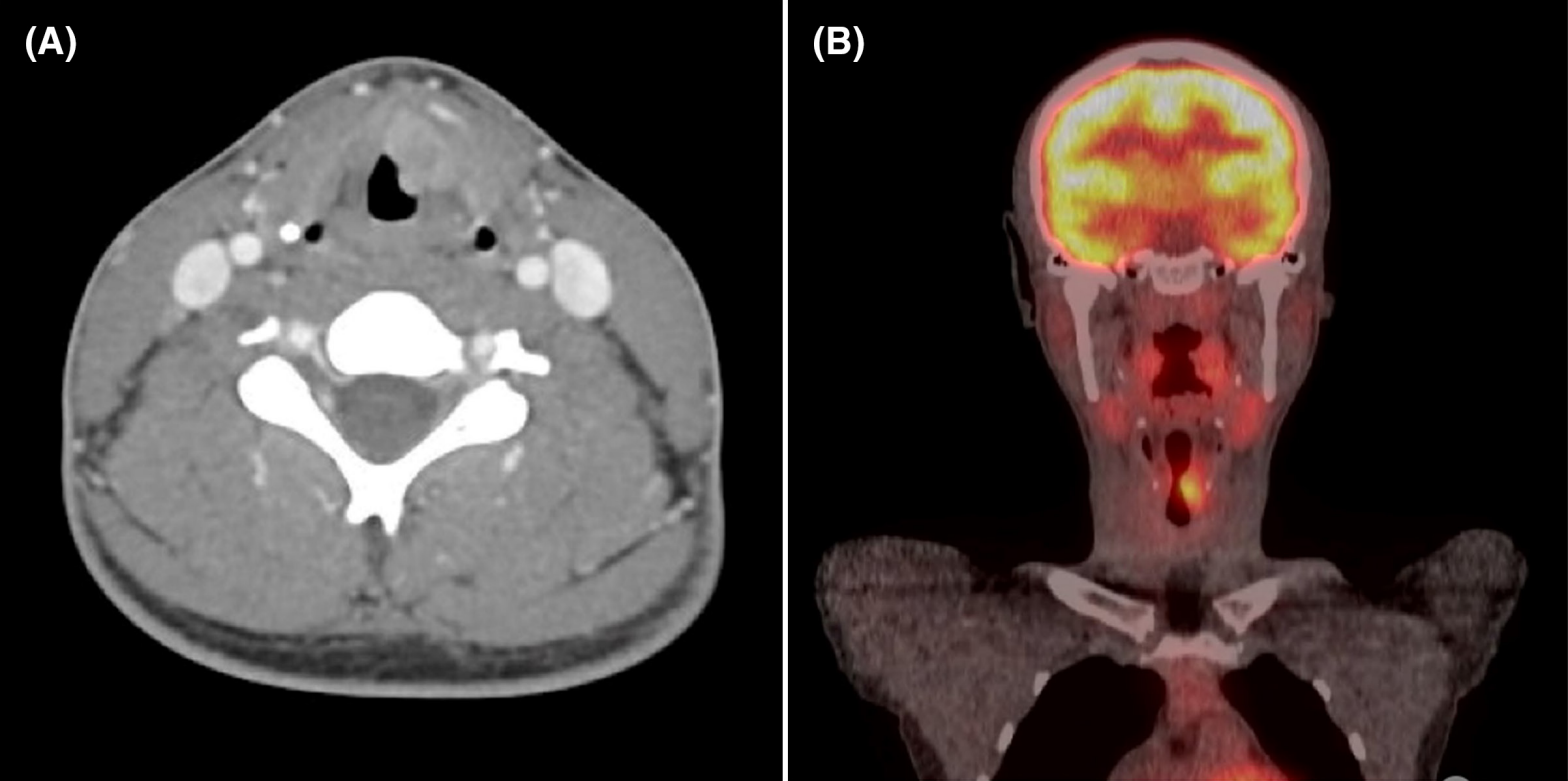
CT with contrast imaging (A) revealed enhancing lesion of the left paraglottic space extending to the anterior commissure corresponding to PET‐FDG avid lesion of the left larynx (B).
